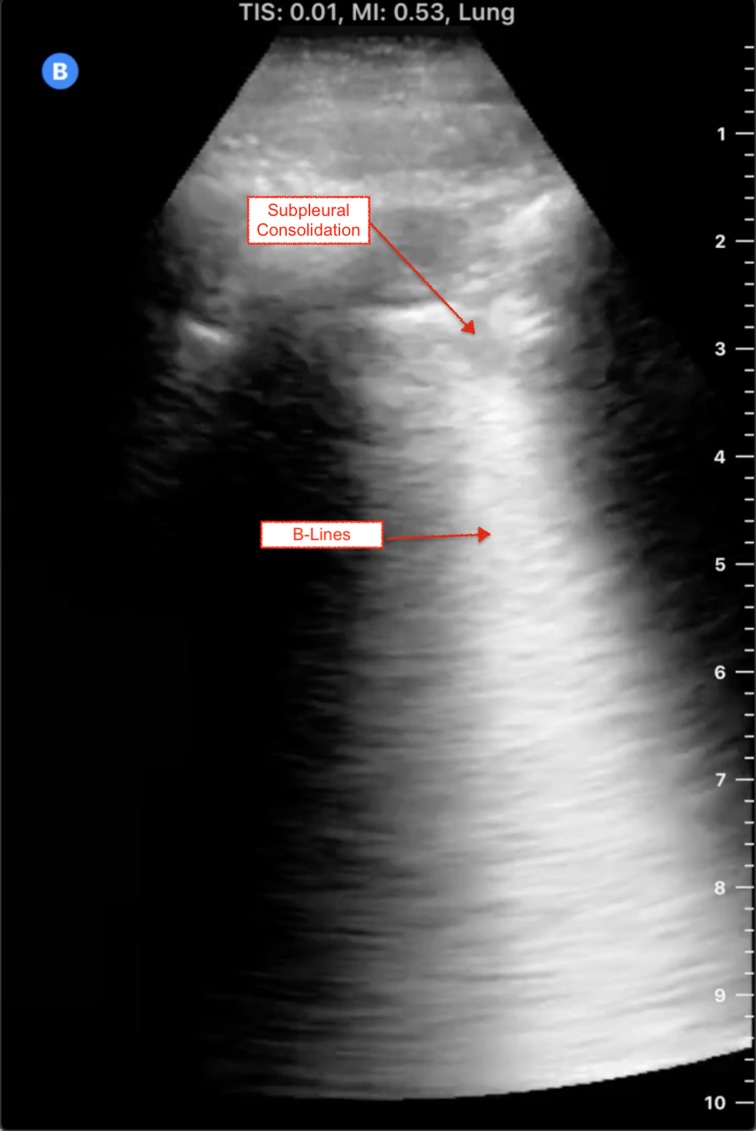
COVID-19-positive patient's lung ultrasound findings.
